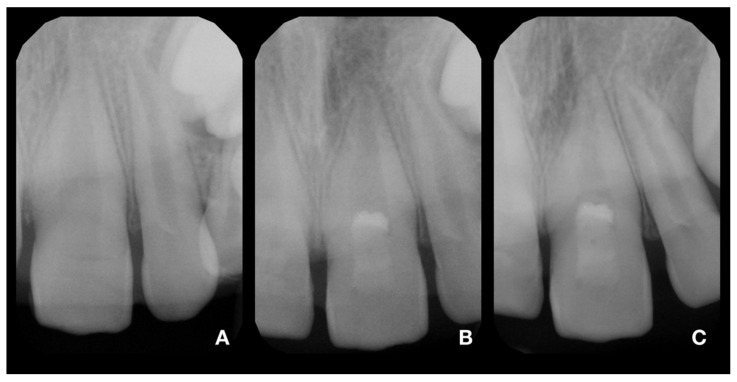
X-rays of tooth 21. (A)—diagnostic X-ray of tooth 21; (B)—postoperative X-ray of tooth 21; (C)—control X-ray at 24-month follow-up. The continuing development of the root and narrowing of the root canal is visible. In addition, the formation of a radiopaque bridge under the calcium silicate cement and the absence of periapical radiolucency are apparent.
